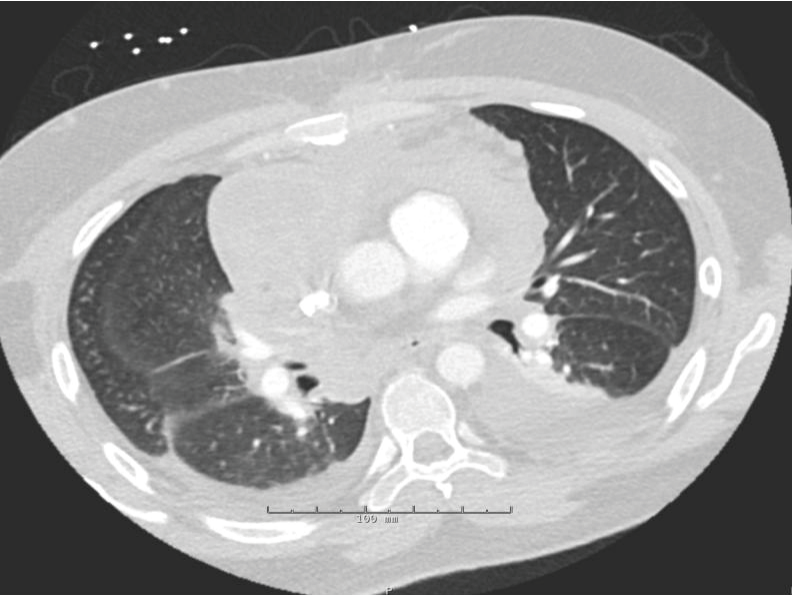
CT chest with angiography: stable anterior mediastinal mass to 6.1 × 5.3 cm (previously noted to decrease in size). Partial bilateral lower lobar atelectasis, interlobular septal thickening increased right anterior and right anterior mammary lymph node, anterior diaphragmatic and anterior mediastinal lymphadenopathy. Increased moderate bilateral pleural effusions [5].
